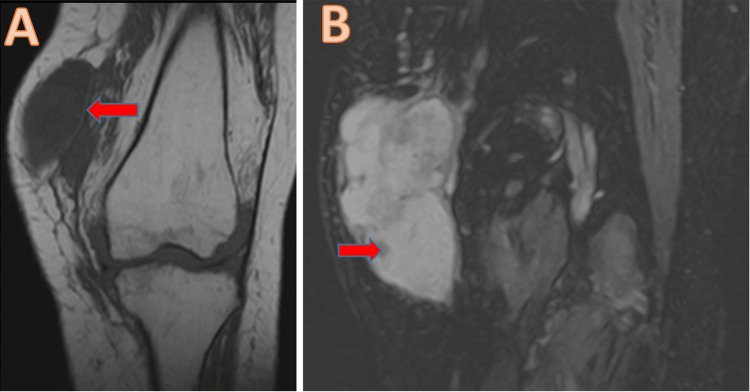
MRI frontal section showing capsule thickening (A) with hemosiderin deposits (B)
